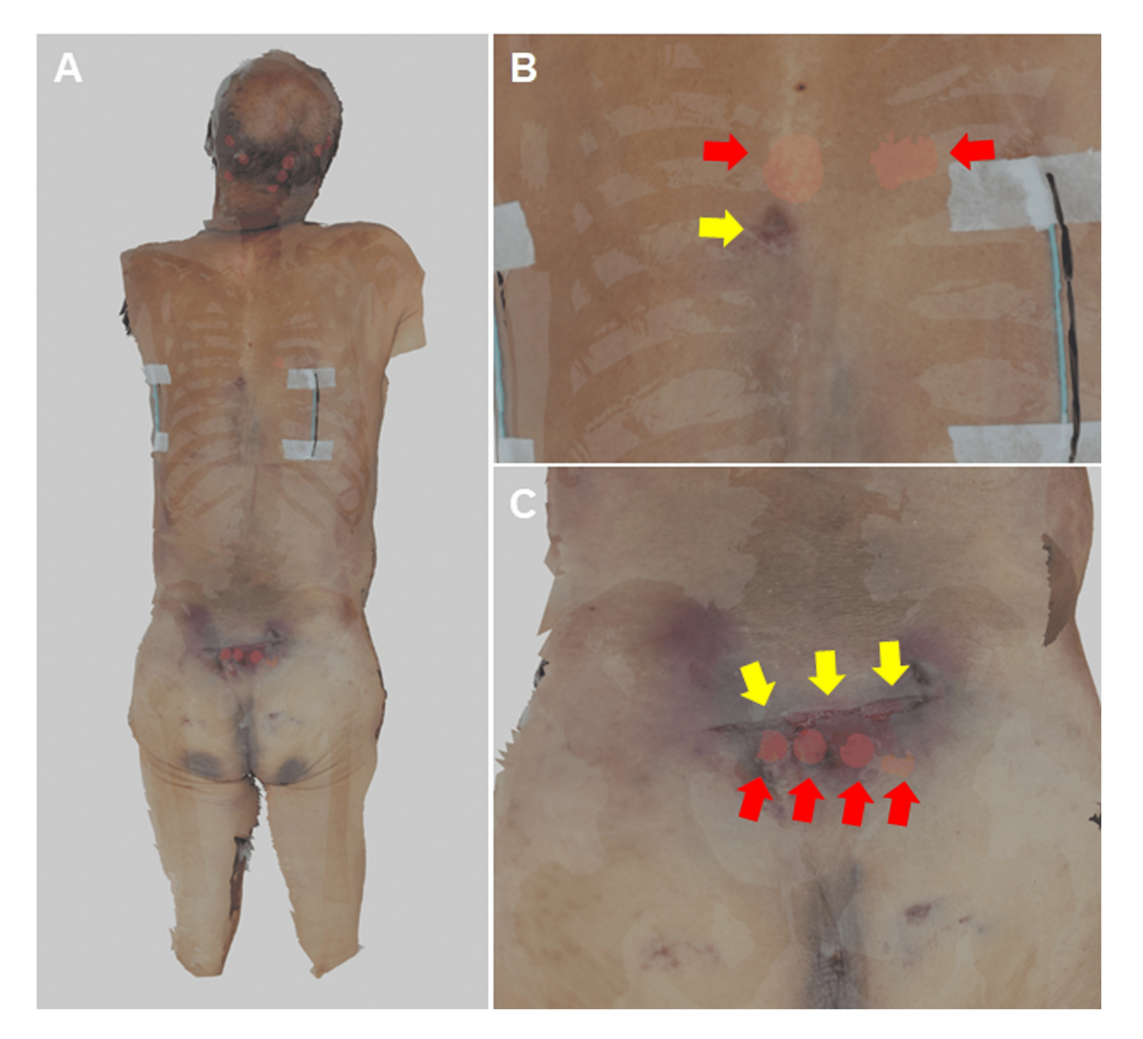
Combined visualization of postmortem CT and photogrammetry models. (A) Overview. The bones and red spheres indicate the fractures beneath the skin surface. (B) Enlarged view of the spinal region. (C) Enlarged view of the sacral region. Red spheres indicating fractures are visible, as indicated by red arrows. Yellow arrows indicate skin injuries.
